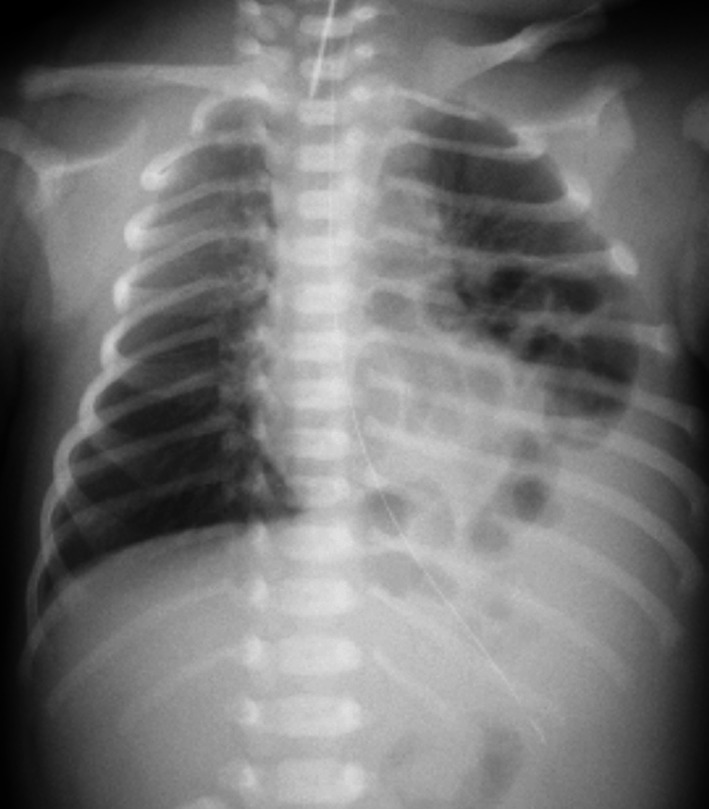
Chest radiograph in case 2. Chest radiograph shows left diaphragmatic hernia
